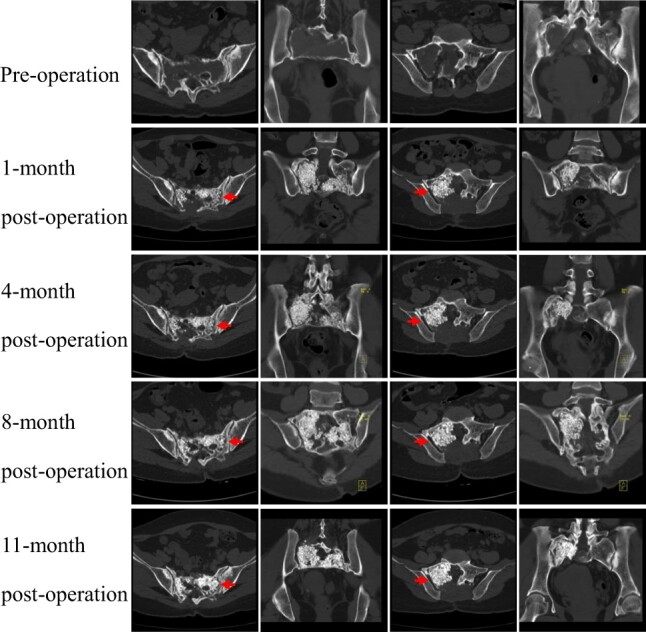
The CT scan of sacrum before and after surgery in Case 2. Left arrow, the CT attenuation value of the artificial bone was gradually increased; right arrow, the cortical rim was rebuilt
